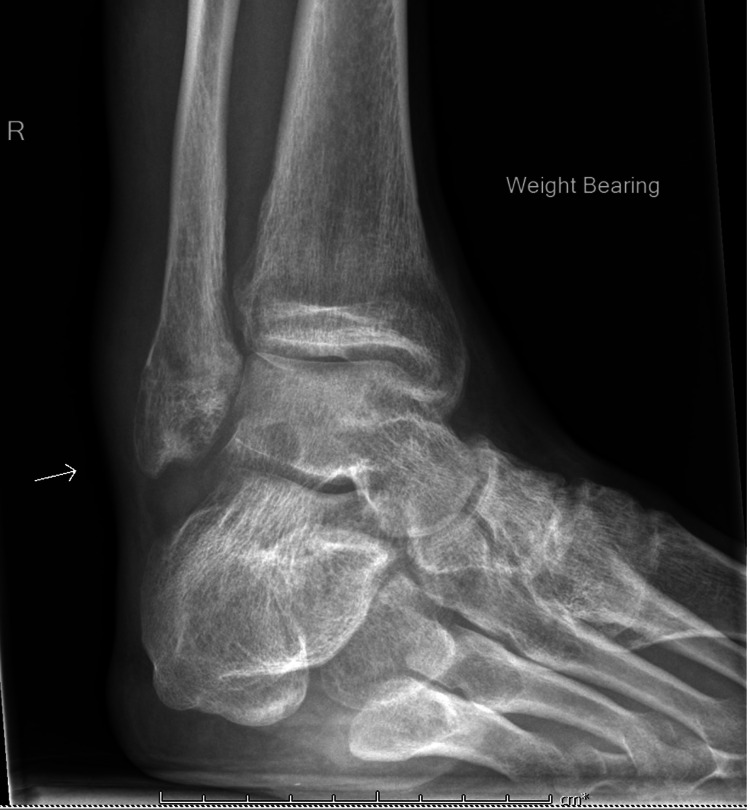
X-ray of the right ankle. Marked soft tissue swelling around the right ankle with marked periarticular osteopenia. There is also a lobulated soft tissue density projecting dorsally to the ankle joint into Kager's fat pad. There is preservation of the joint space width.
